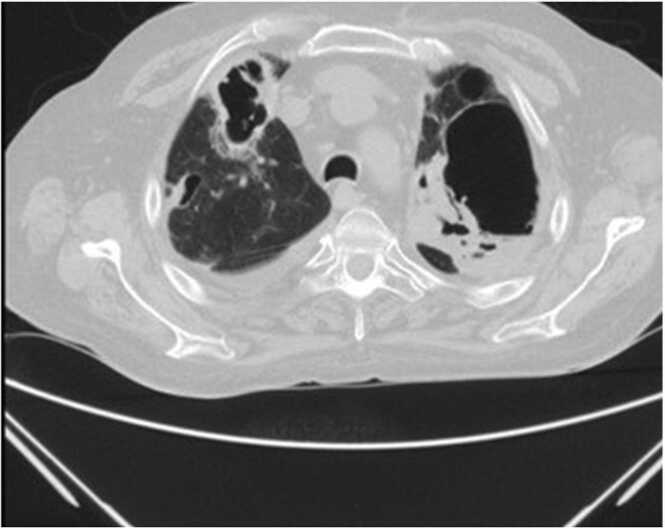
Chest CT scan showing progression of ground glass opacities into consolidation, and large cavitary lesions in bilateral lung fields.
